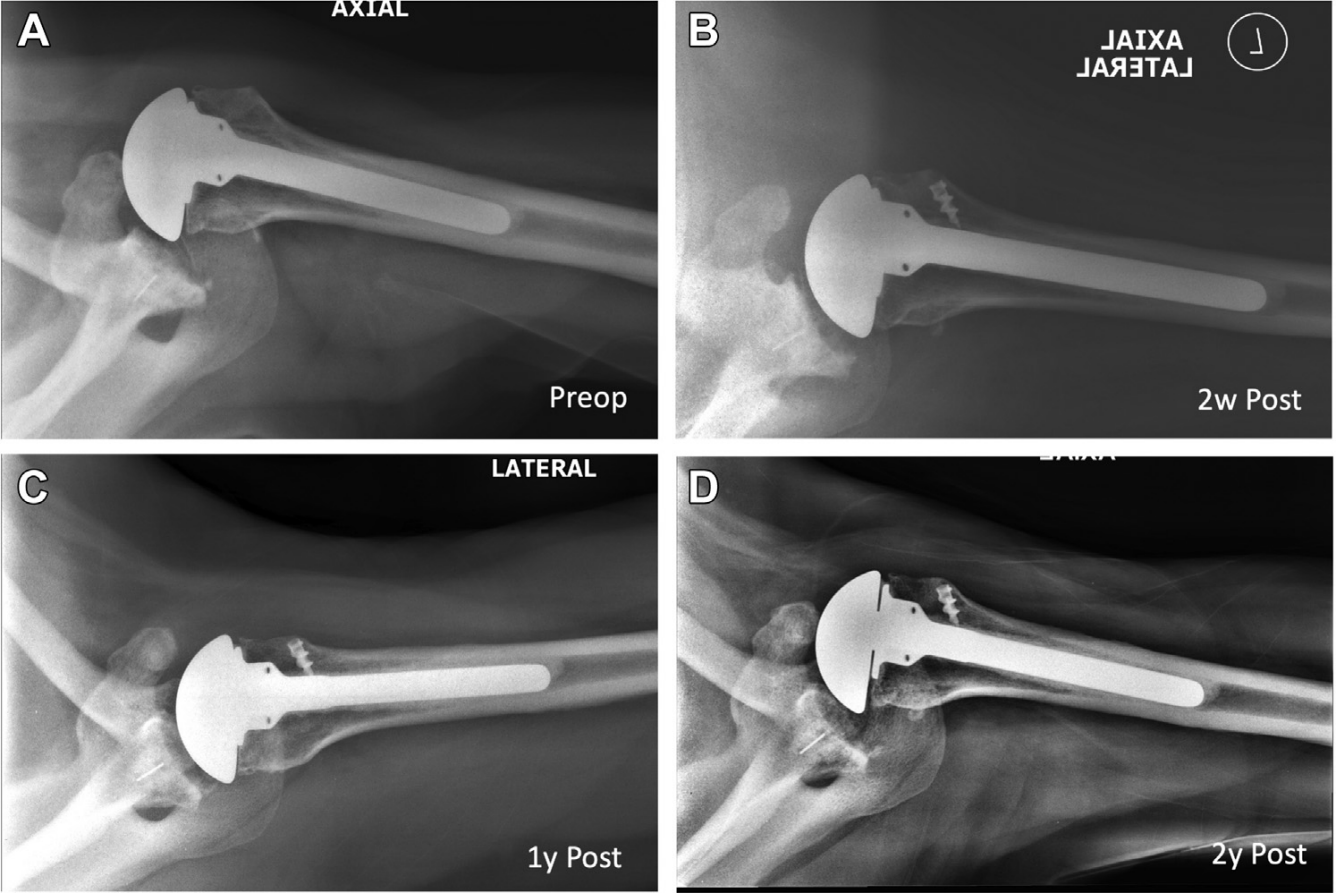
(A) Axillary shoulder radiographs from patient 2 demonstrating anterior translation of the humeral head on the glenoid preoperatively. (B) This alignment is improved on radiographs obtained 2 weeks postoperatively from pectoral major muscle transfer. (C) Continued alignment was noted at approximately 1 year postoperatively. (D) Anterior translation was discovered to recur on radiographs obtained approximately 2 years postoperatively. Patient continued to be pain free with preserved function despite radiographic instability.
